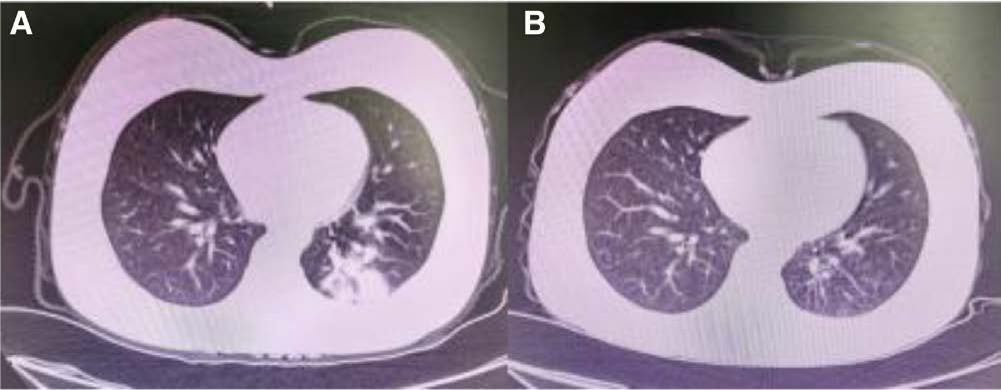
Computed tomography scan. (A) CT images before receiving anti-infection treatment with piperacillin-tazobactam. (B) CT images of patients experiencing fever again.
